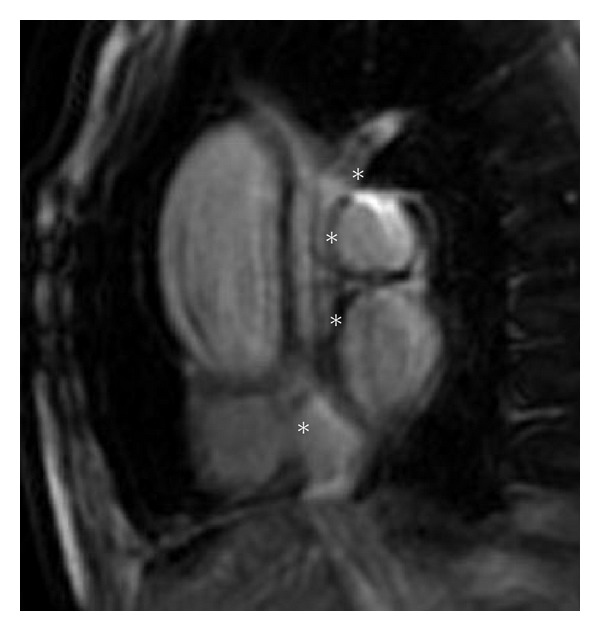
MRI showing the entire course of the thrombus (*) from the left brachiocephalic vein, SVC, and finally to the right atrium.
